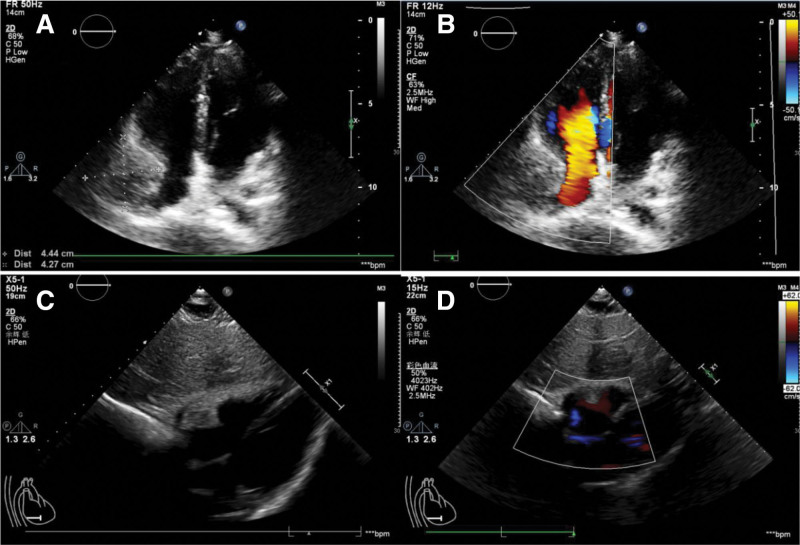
(A) One-month postoperative cardiac echocardiogram showing a slightly hypoechoic mass measuring approximately 44 × 43 mm on the lateral wall of the right atrium. The mass has a relatively regular shape, ill-defined borders, and heterogeneous internal echogenicity. (B) Color Doppler imaging reveals no significant blood flow signal within the mass. (C, D) A slightly hypoechoic mass is observed at the anterolateral aspect of the pericardium on the subxiphoid view, with compression of the superior vena cava and the right atrium. The margins of the lesion are ill-defined.
